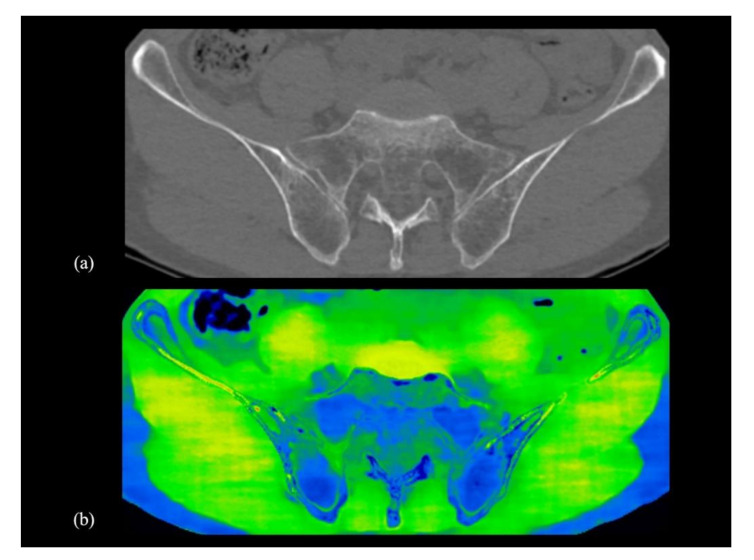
Axial view of the pelvic bones of conventional CT image and water-HAP image in an oncologic patient without bone metastasis in his 70s. (a,b) The pelvic bone’s water density is comparable to that of subcutaneous adipose tissue and lower symmetrically than that of skeletal muscle. This finding suggests the absence of bone tumours. HAP; Hydroxyapatite.
